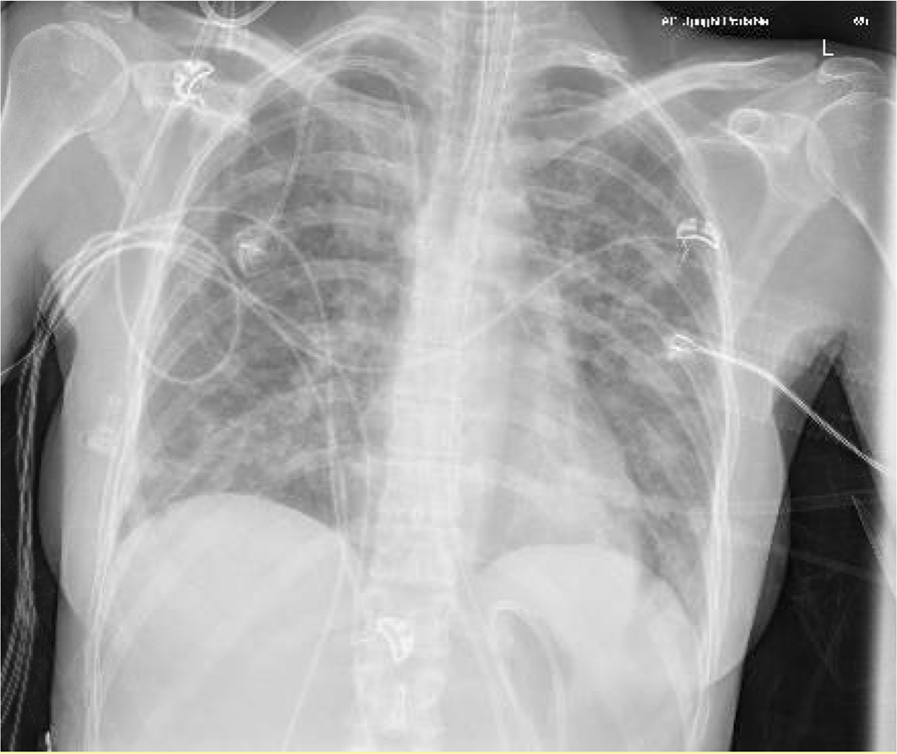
Repeated chest X-ray 5 days later, with improving bilateral opacities
